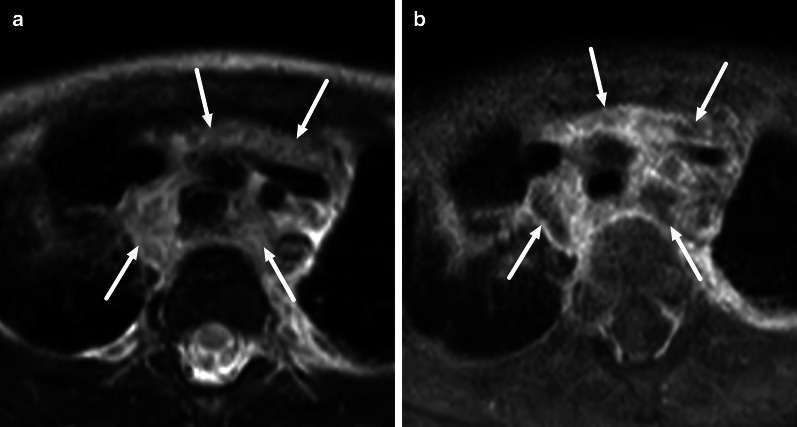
Mediastinal extension of abscesses (arrows) on axial T2-weighted (a) and fat-suppressed post-contrast T1-weighted (b) images in an infant. Note the somewhat degraded image quality at this level. The patient underwent thoracoscopy 3 days after MRI because the condition worsened during medical management
